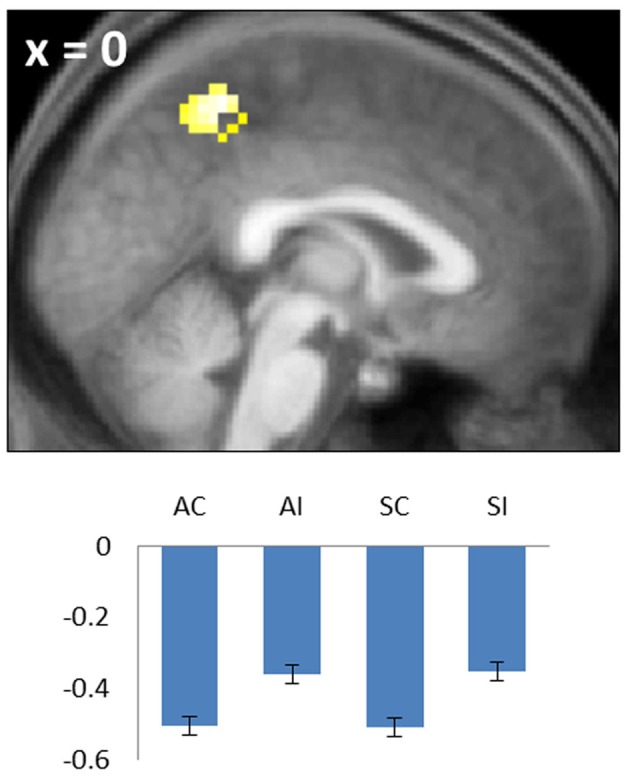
Negative common subsequent memory effect in the medial parietal cortex (precuneus). Bar plot shows the peak parameter estimates for the four conditions of interest. Results are overlaid onto a sagittal section of the across-subjects mean T1-weighted anatomical image.
